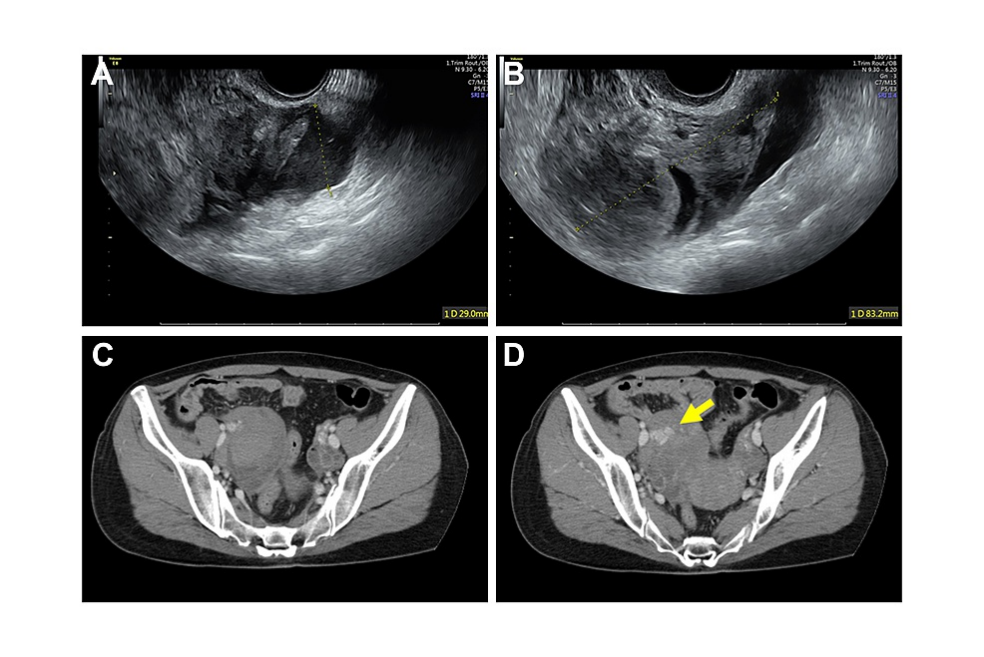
Photographs of transvaginal ultrasound and images of computed tomography (CT) of the patients with acute abdomen after oocyte retrieval at hospital transport. Echo-free space in the Douglas’s pouch (A) and swelling of the right adnexa (B) are observed. CT images of right adnexal hematoma (C) and contrast medium leaking from a blood vessel (D, arrow).
